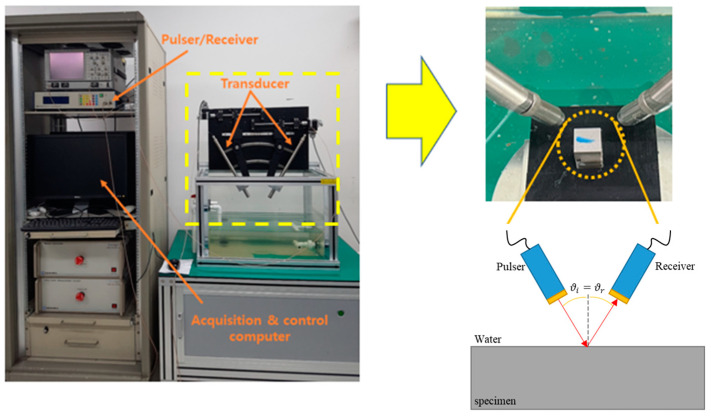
Ultrasonic immersion testing experiment setup with pitch-catch method.
